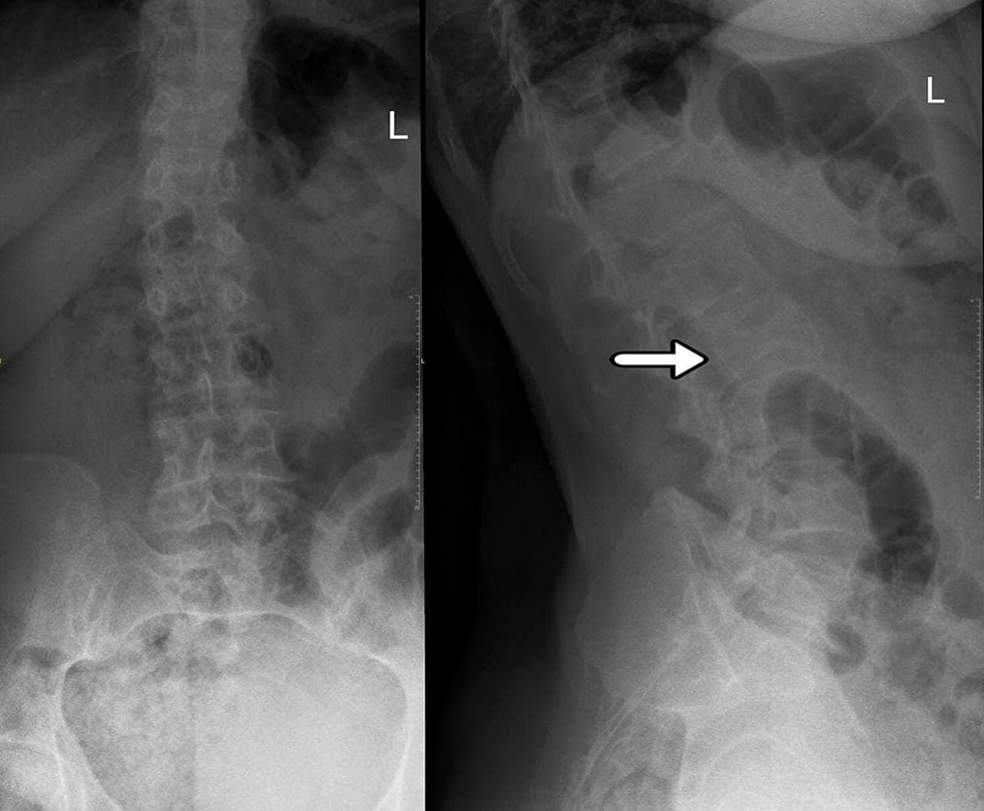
X-ray of the lumbar spine; AP and lateral views Showing L2 compression, with no obvious fracture
